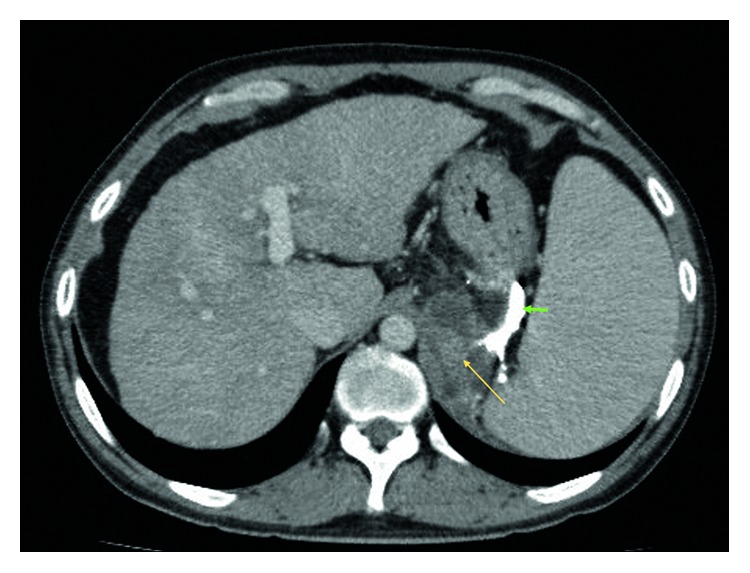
Computed tomography view of the abdomen (in axial view). The hypodense collection (yellow arrow) behind the stomach corresponds to the retrogastric abscess. The residual N-butyl-2-cyanoacrylate in the perigastric region is more explicit in this view (green arrow).
